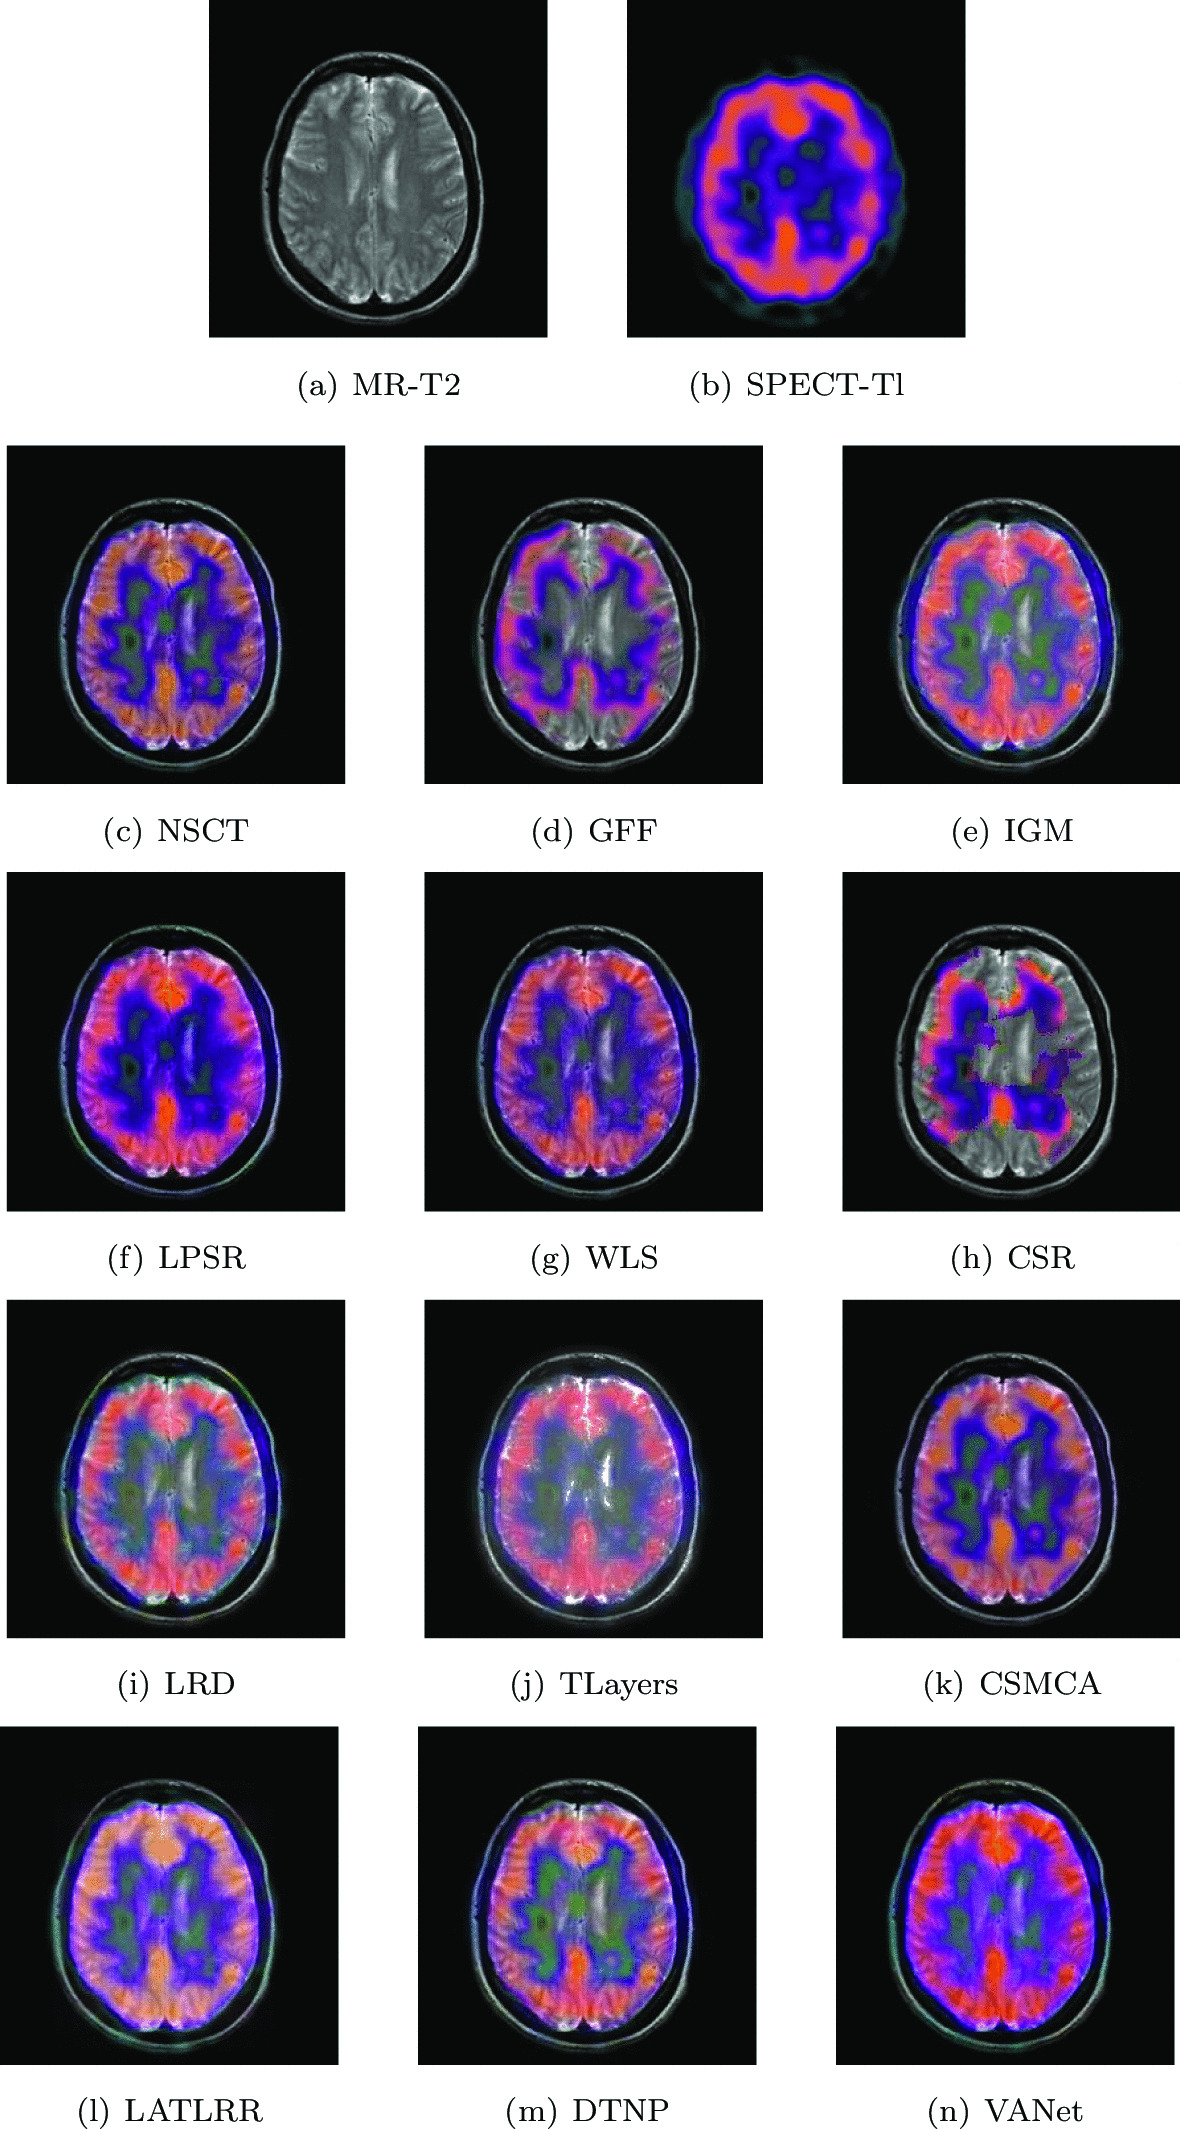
The second set of fused MRI-SPECT images from 9 methods on hypertensive encephalopathy
